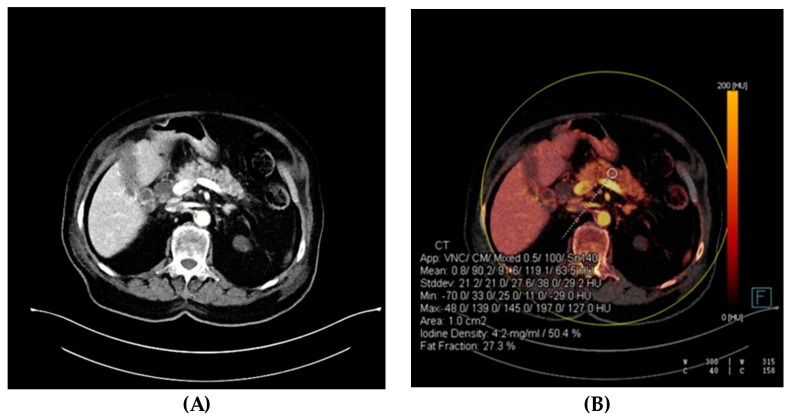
Dual-energy computed tomography (DECT) quantification. Axial images of a 91-year-old female patient with acute interstitial edematous pancreatitis. APACHE II = 13, mCTSI = 2. (A) Blended greyscale image showed mild gland enlargement. (B) Iodine overlay image with region-of-interest (ROI) and quantification of attenuation values, iodine density, and fat fraction. APACHE II, Acute Physiology and Chronic Health Evaluation II; mCTSI, modified CT severity index.
